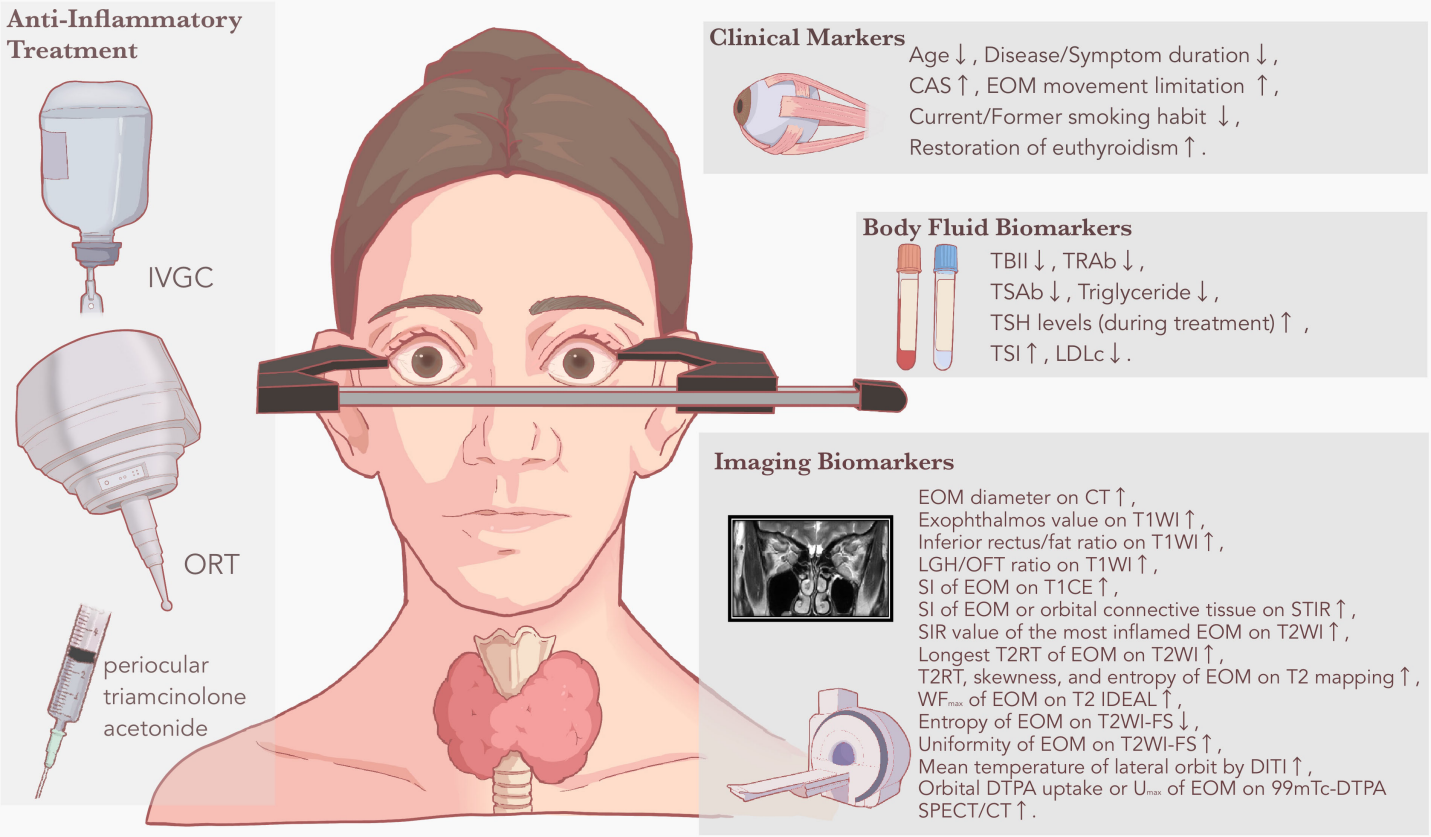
Clinical and biological markers for predicting anti-inflammatory treatment response in TED. Based on the literature review, common anti-inflammatory treatment methods identified with predictive markers or prediction models include IVGC, ORT, and periocular triamcinolone acetonide. Based on different derivations, predictive markers are displayed as clinical markers, body fluid biomarkers and imaging biomarkers, respectively. A rising arrow suggests that the predictive marker is positively related to treatment response to anti-inflammatory treatment, while a falling arrow suggests that the predictive marker is negatively related to treatment response to anti-inflammatory treatment. CAS, clinical activity score; CT, computed tomography; DITI, digital infrared thermal imaging; DTPA, diethylenetriaminepentaacetic acid; EOM, extraocular muscle; FS, fat-suppressed sequences; IVGC, intravenous glucocorticoid; LDLc, low-density lipoprotein cholesterol; LGH, lacrimal gland herniation; MRI, magnetic resonance imaging; OFT, orbital fat thickness; ORT, orbital radiation therapy; SI, signal intensity; SIR, signal intensity ratio; SPECT, single-photon emission computed tomography; STIR, short time of inversion recovery; T1WI, T1-weighted sequences; T2RT, T2 relaxation time; T2WI, T2-weighted sequences; TBII, thyrotropin-binding inhibitory immunoglobulin; TED, thyroid eye disease; TRAb, thyrotropin receptor antibody; TSAb, thyroid-stimulating antibody; TSH, thyroid-stimulating hormone; TSI, thyroid stimulating immunoglobulin; U_max_, maximum uptake ratio; WF_max_, maximum water fraction of extraocular muscle scintigraphy.
